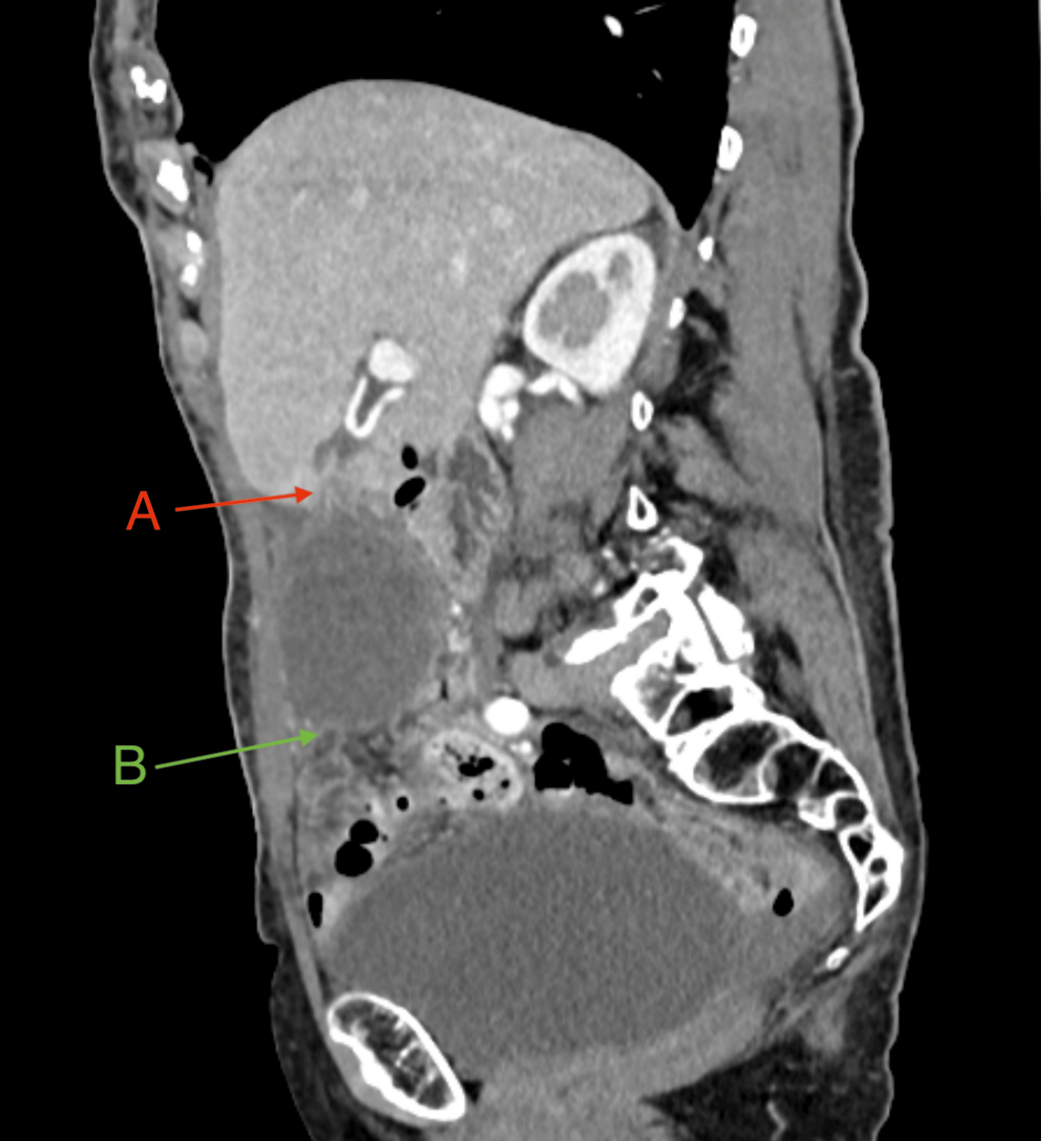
CT abdomen, sagittal plane (early arterial phase). (A) Filling defect of the cystic artery (*arteria cystica*). (B) Gallbladder hydrops with pericystic fluid.
